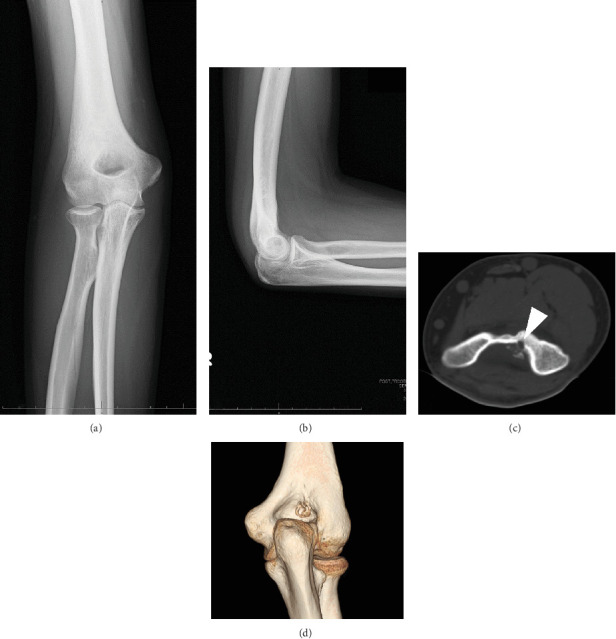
Preoperative plain radiography and computed tomography (CT). (a) Anteroposterior and (b) lateral views. No remarkable findings. (c) Axial and (d) three-dimensional CT images show the nidus (white arrowhead) in the distal humerus and reactive bone at the olecranon fossa.
